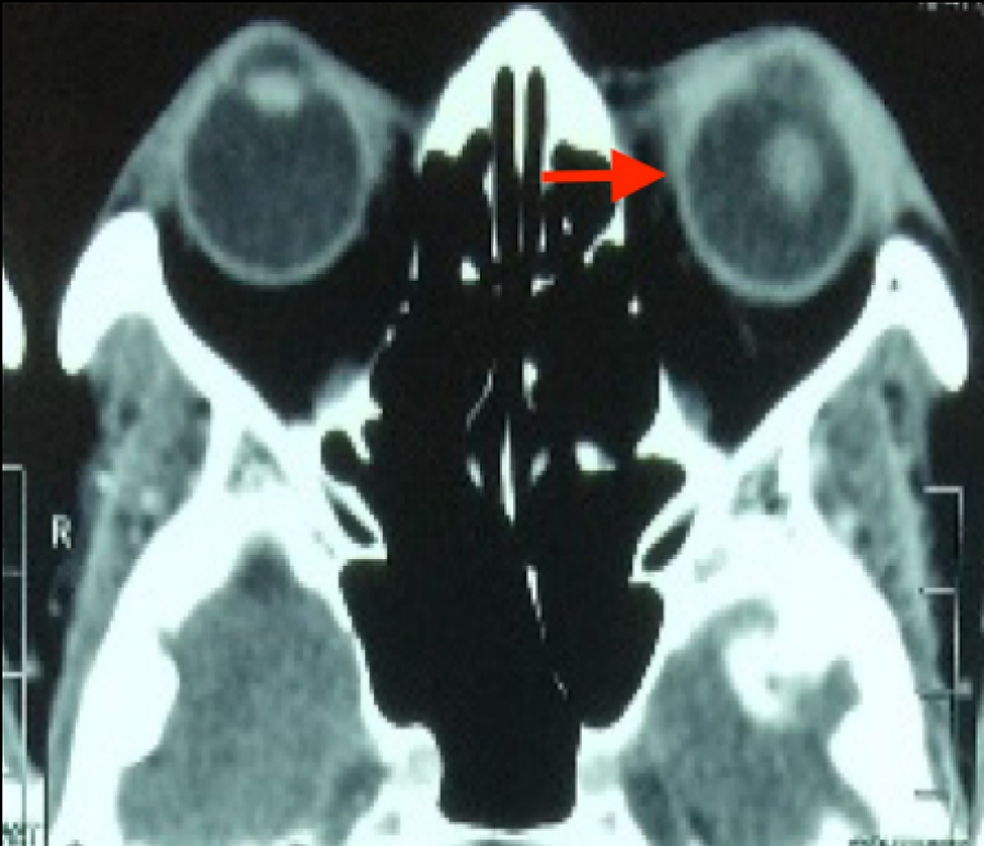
CECT orbit shows left periorbital soft tissue thickening and enhancement suggestive of inflammatory/infective changes. There is also lens dislocation probably due to the breakdown of zonular fibers by Klebsiella pneumoniae. CECT: contrast-enhanced computed tomography
